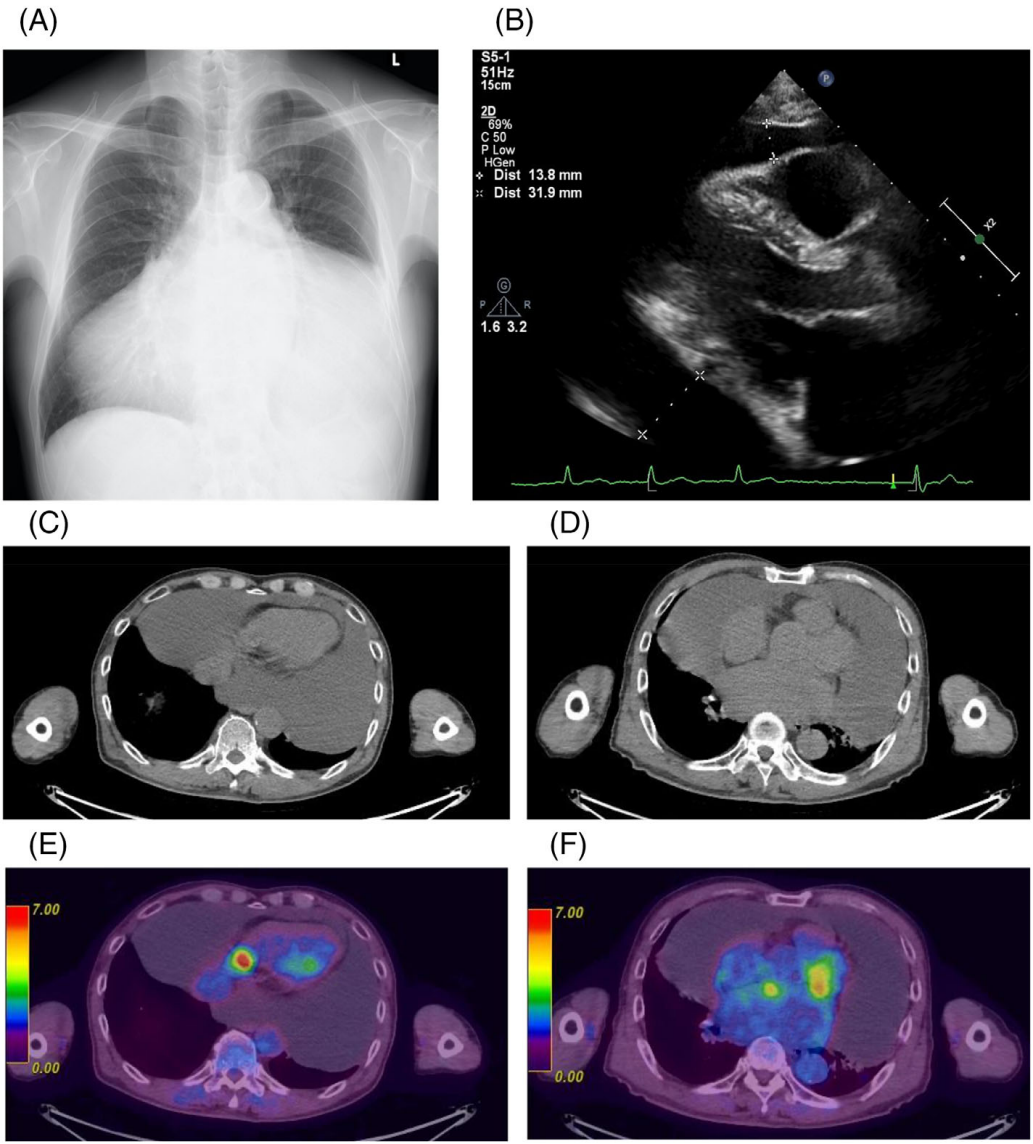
Chest radiography (A) and percutaneous echocardiography (B) taken at initial presentation. Notable cardiac enlargement and massive pericardial effusion are observed. Chest computed tomography (CT) (C, D) and ^18^F‐fluorodeoxyglucose (FDG) positron emission tomography‐CT (E, F) scans at initial presentation. The image shows increased uptake of FDG on the right ventricular caudal side (C and E), around the pulmonary artery and around the root of the aorta (D and F).
